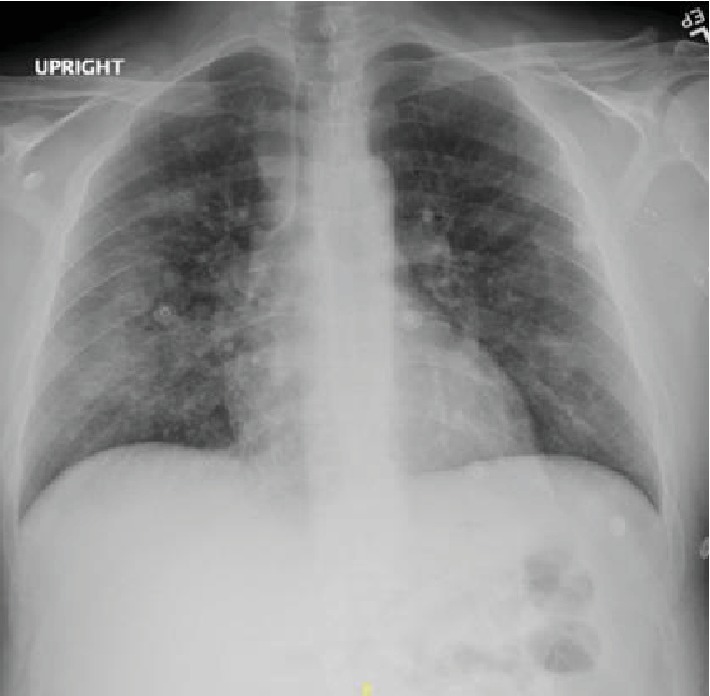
Chest X-ray showing diffuse bilateral infiltrates, more pronounced on the right side.
